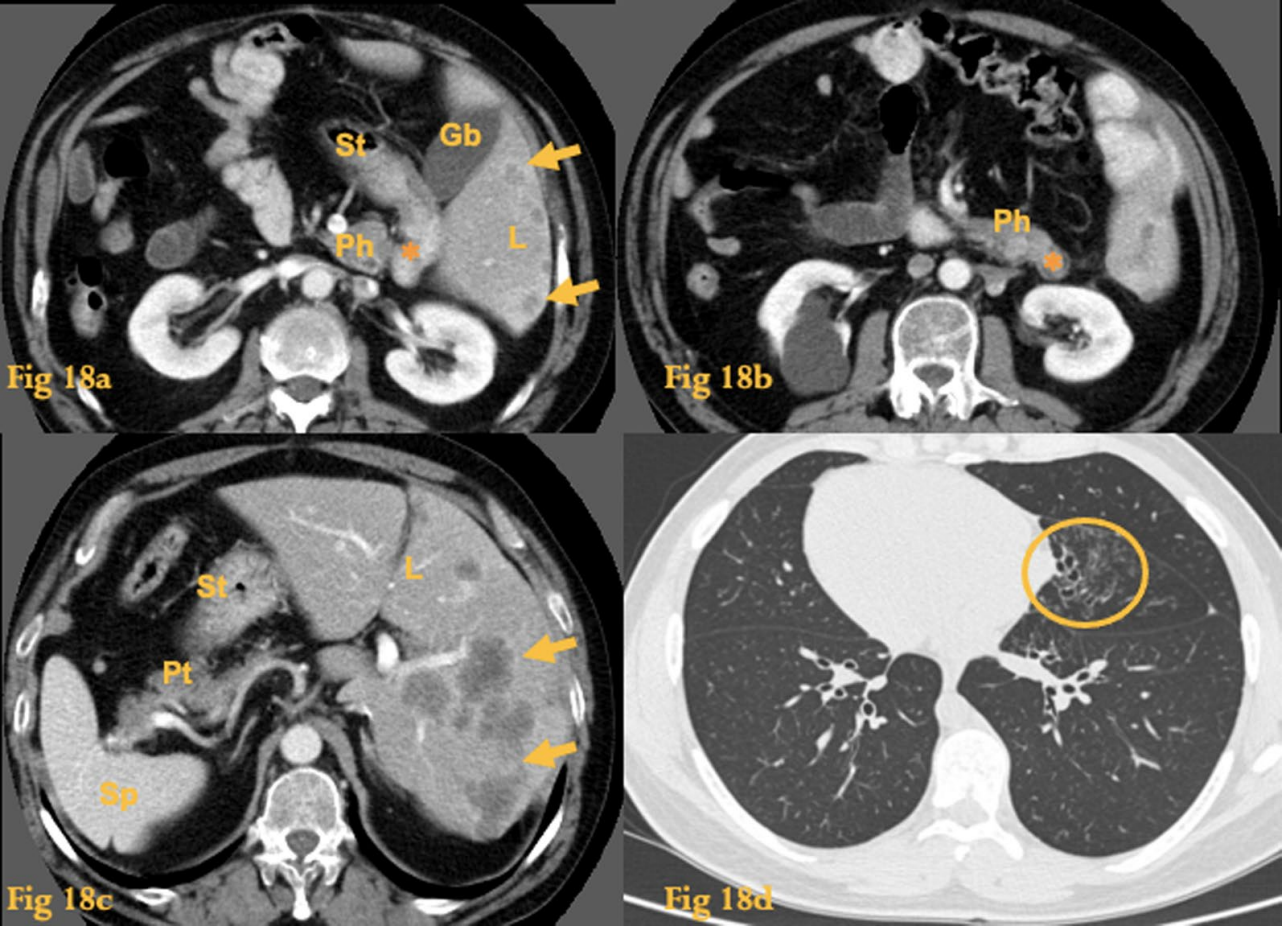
Kartagener syndrome. Abdominal CT of a patient with situs inversus. There are hypodense liver lesions (arrows) corresponding to metastases from colon cancer (a, c). The duodenum accompanies the mirror image of the other abdominal organs. Asterisk = descending duodenum. L = liver; S = spleen; St = stomach; Ph = head of the pancreas; Pt = tail of the pancreas; Gb = gallbladder. Thoracic CT of another patient (d) with situs inversus and Kartagener syndrome shows bronchiectasis in the middle lobe of the left lung (circle)
